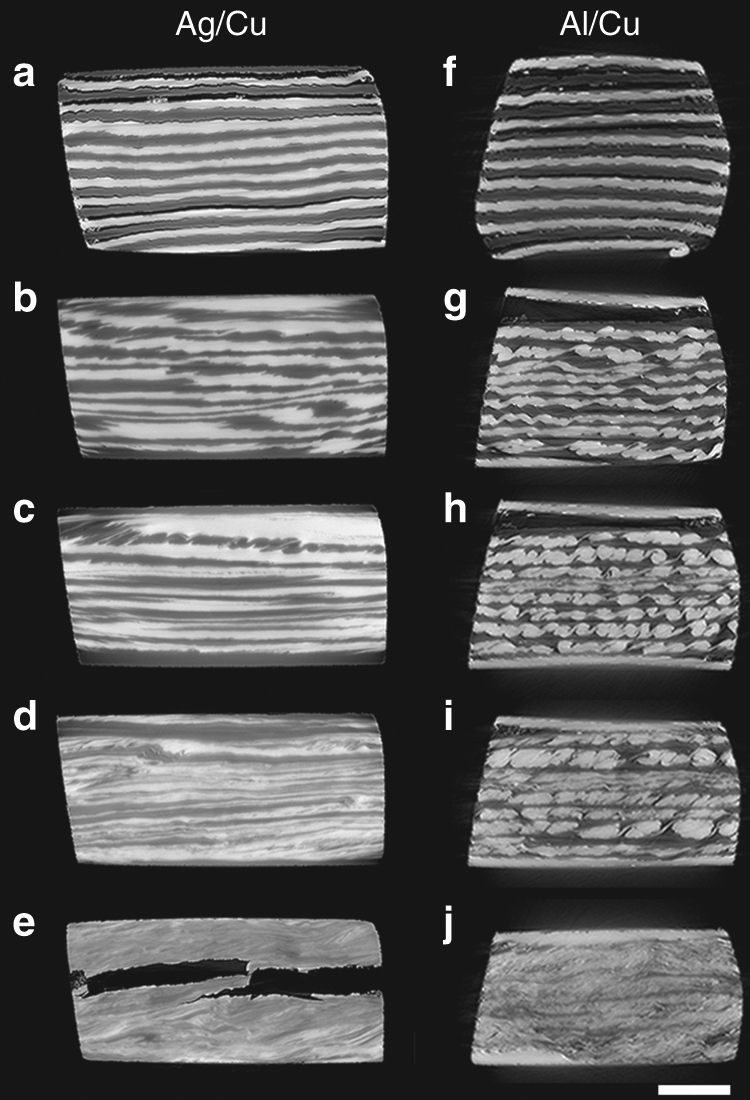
Morphological evolution of multilayers upon shearing acquired by 3D X-ray synchrotron tomography. a–e Selected snapshots of Ag/Cu-multilayer sample that had been shear-deformed parallel to the layers at 1 r.p.m. for five HPT revolutions, corresponding to a maximum strain of 393. The brighter phases are Ag. Here, the Cu layers predominantly delaminate before mixing at micrometer scale takes place. See Supplementary Movie 1 for the entire deformation sequence. f–j Selected snapshots of the Al/Cu-multilayer sample, shear-deformed parallel to the layers at 1 r.p.m. for three HPT revolutions, corresponding to a maximum strain of 236. The brighter phases are Cu. Here, the Cu layers initially fold in a quasi-regular manner and subsequently evolve into periodic vortices, before mixing at micrometer scale takes place. See Supplementary Movie 2 for the whole sequence. Note that in both sequences, the overall strain increases when going from the top to the bottom of each panel. The scale bar is 200 µm. The dark area within e is a crack and it occurred after HPT deformation, during the cutting of the disk for tomography examination
